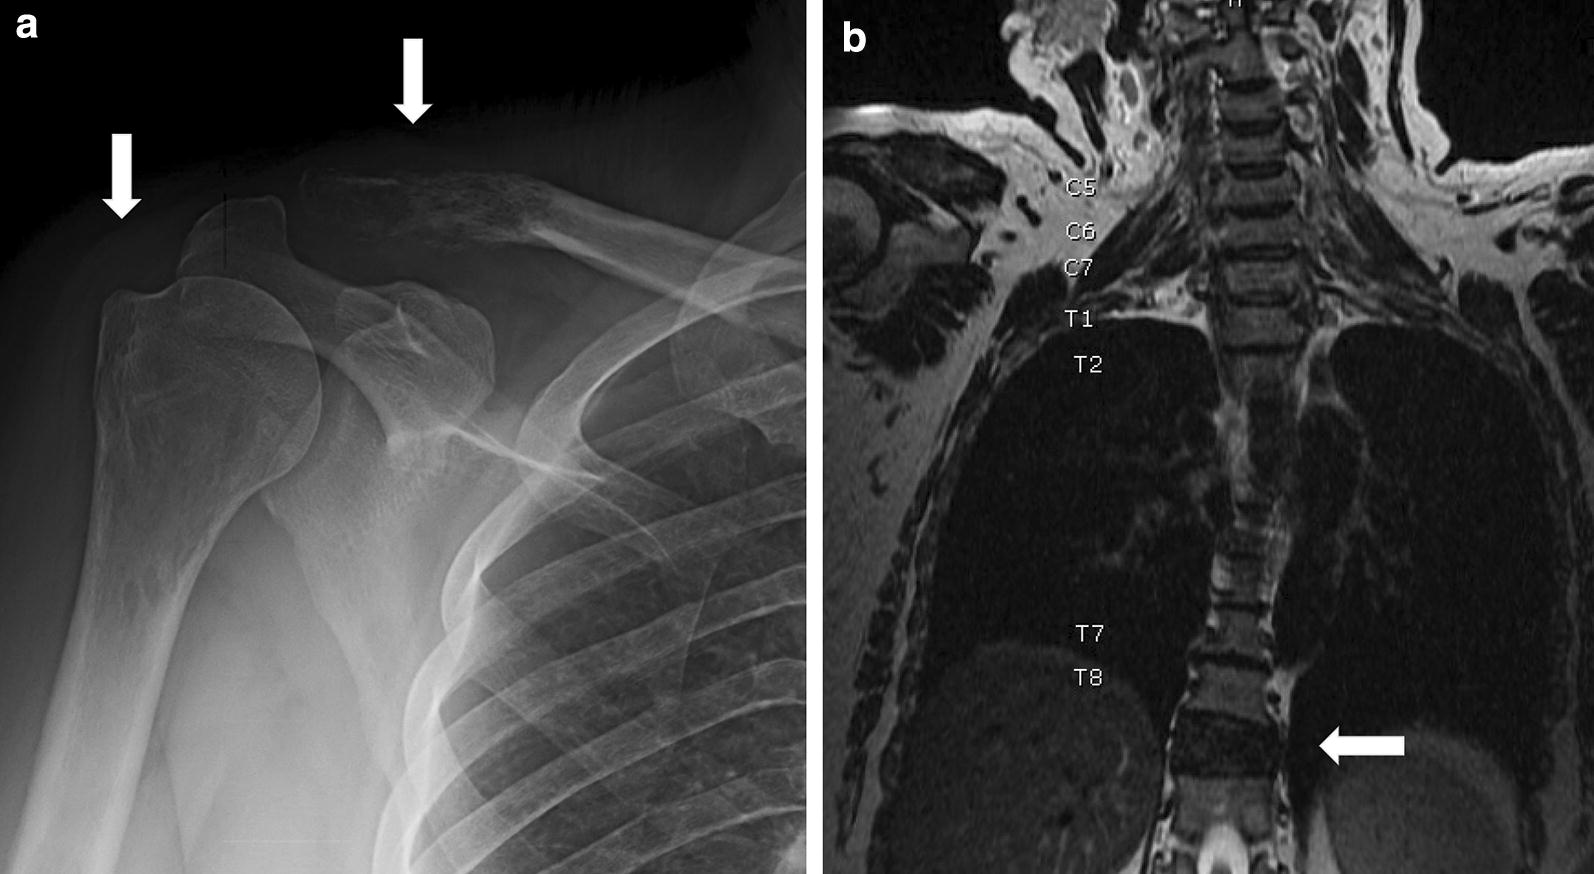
Initial radiography of Lesions. a Plain film radiography showing the lytic lesions present in the right distal clavicle and humeral head (arrows). b T1 MRI of the chest showing the T9 pathologic fracture (arrow)
